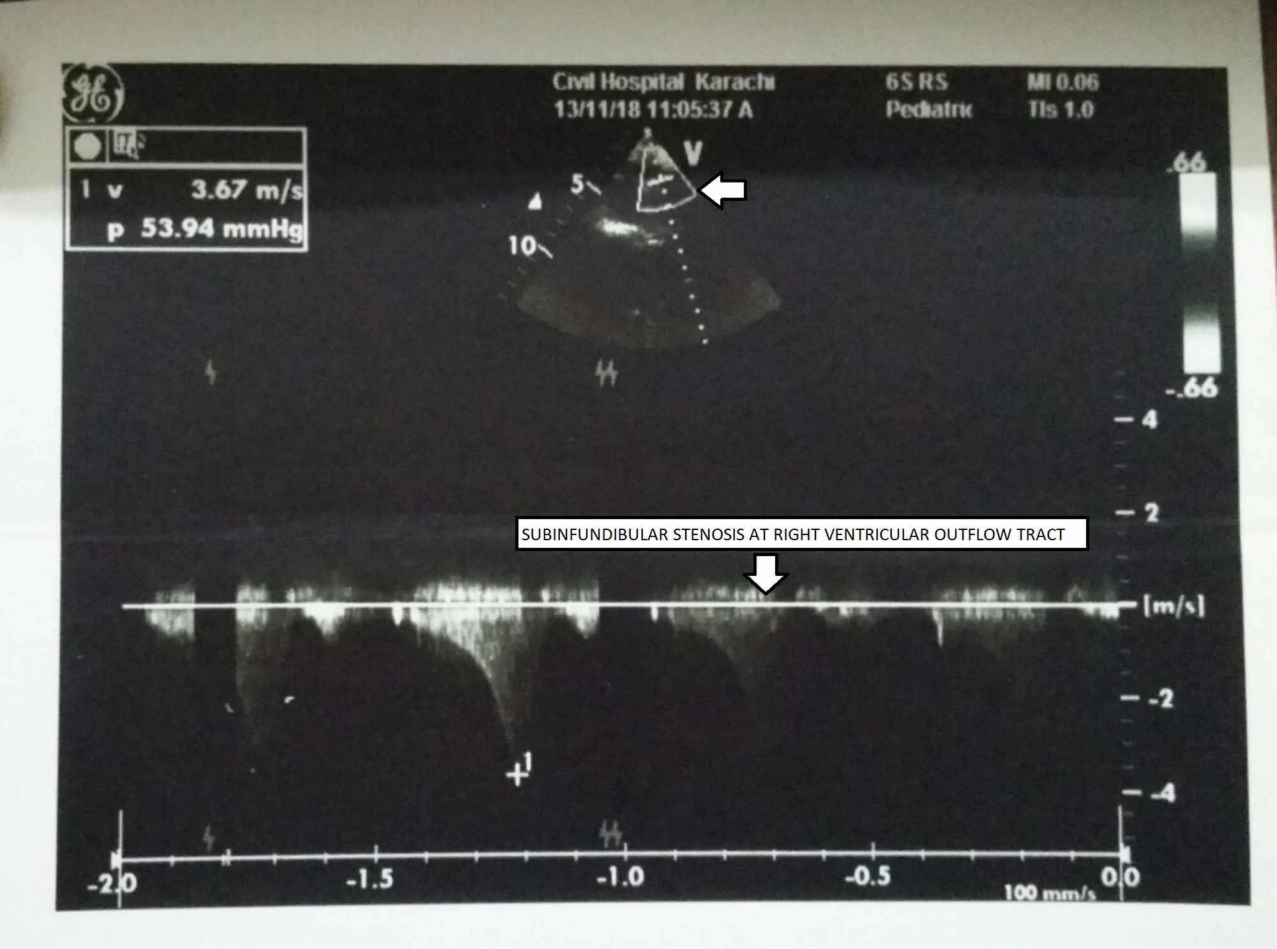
Transthoracic echocardiogram showing sub-infundibular stenosis at the right ventricular outflow tract
